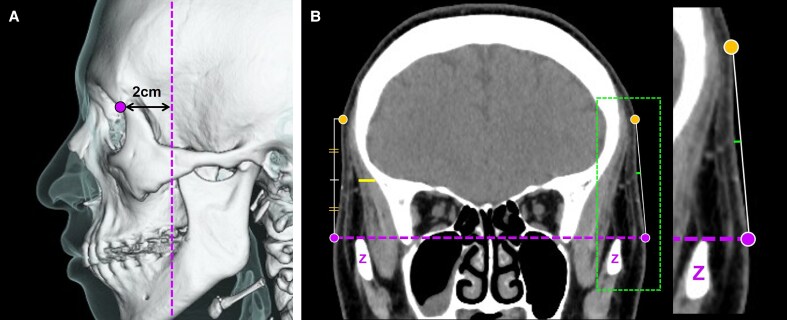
(A) To evaluate the thickness of the temporal muscle and the degree of the temple contour, coronal computed tomography (CT) images were reconstructed 2 cm dorsal to the lateral surface (indicated by a purple circle) of the superior orbital rim, along the purple dashed lines. Measurements were obtained on both the control and the neuromuscular electrical stimulation-applied sides. (B) The thickness of the temporal muscle (length of the yellow line) was measured at the level of the midpoint of a line connecting the surface of the maximal head circumference (indicated by a yellow circle) and the superior margin of the zygomatic arch (indicated by purple circles and dashed lines). In the same coronal CT image (magnified in the right panel), a reference line was drawn connecting the surface of the maximal head circumference and the superior margin of the zygomatic arch. The perpendicular distance (green line) from this reference line to the surface of the temple area was defined as the “degree of the temple contour”.
